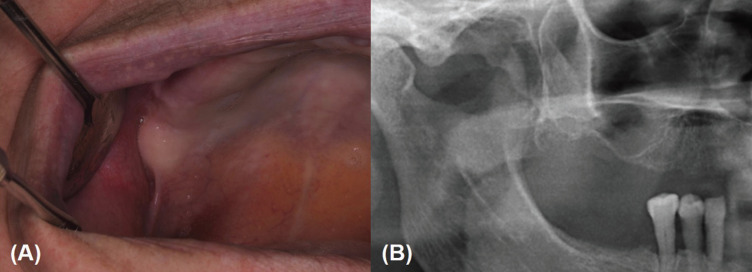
Complete healing of mucosal alveolar mucosa (A) and bone integrity on panoramic radiograph (B) in a follow-up of 6 months after the end of the treatment sessions.
